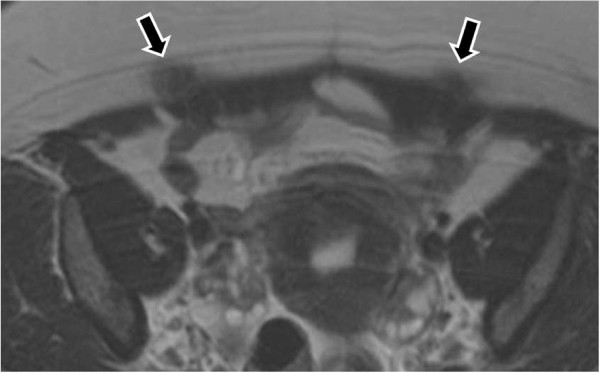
MR images demonstrate foci of endometriomas at the sites of the section [T-2 w.i].
